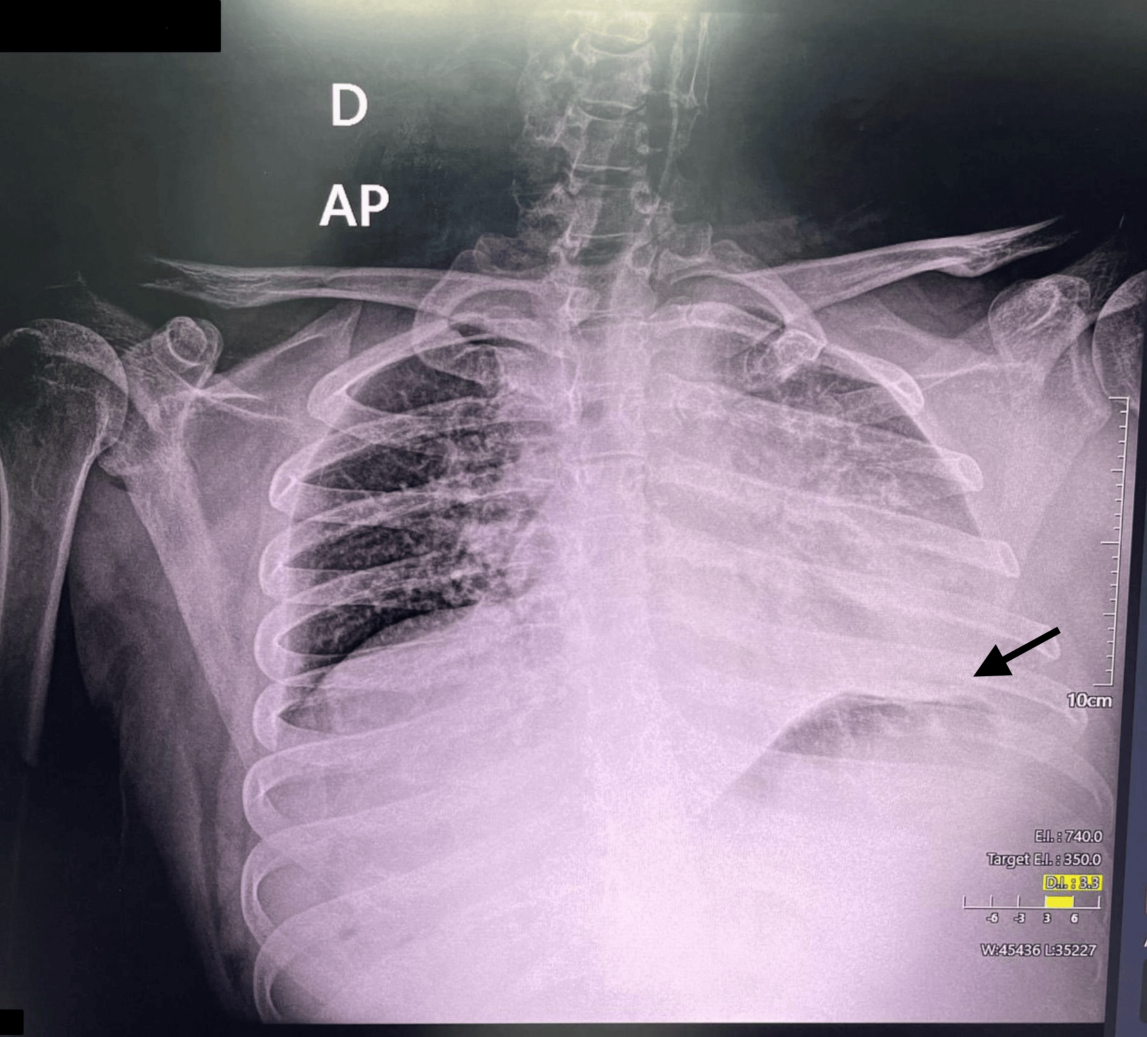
Initial chest X-ray showing a left basal-predominant interstitial infiltrate. AP: anteroposterior; D: derecho (right).
